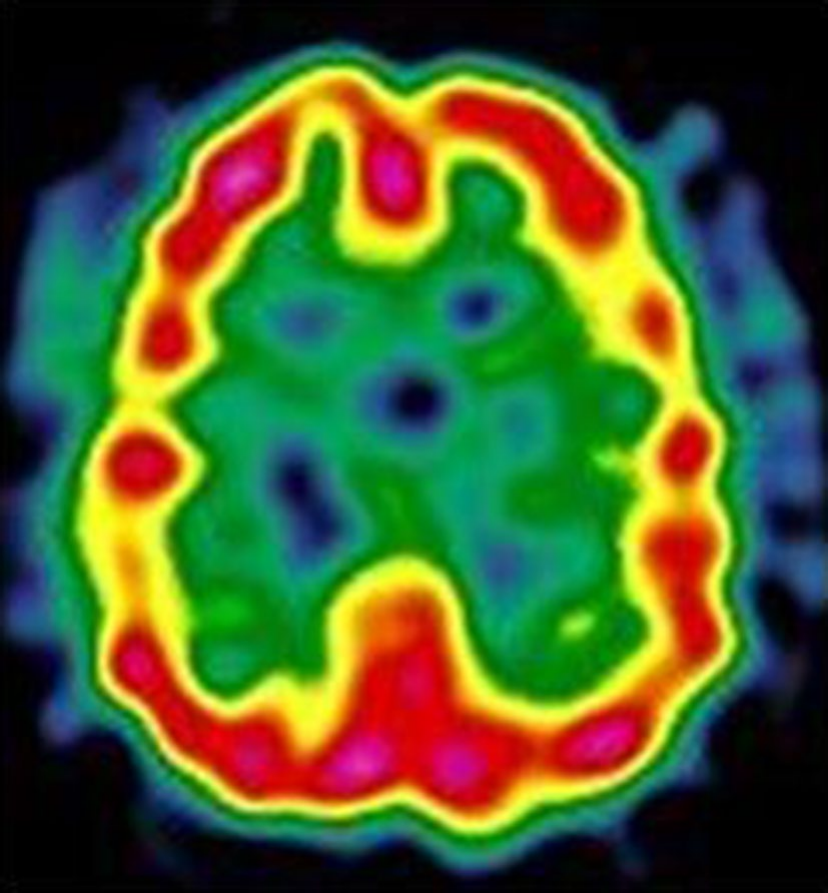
Case # 3. ^99m^Tc-HMPAO brain SPECT: non-homogeneous tracer uptake in the cortex of both hemispheres
